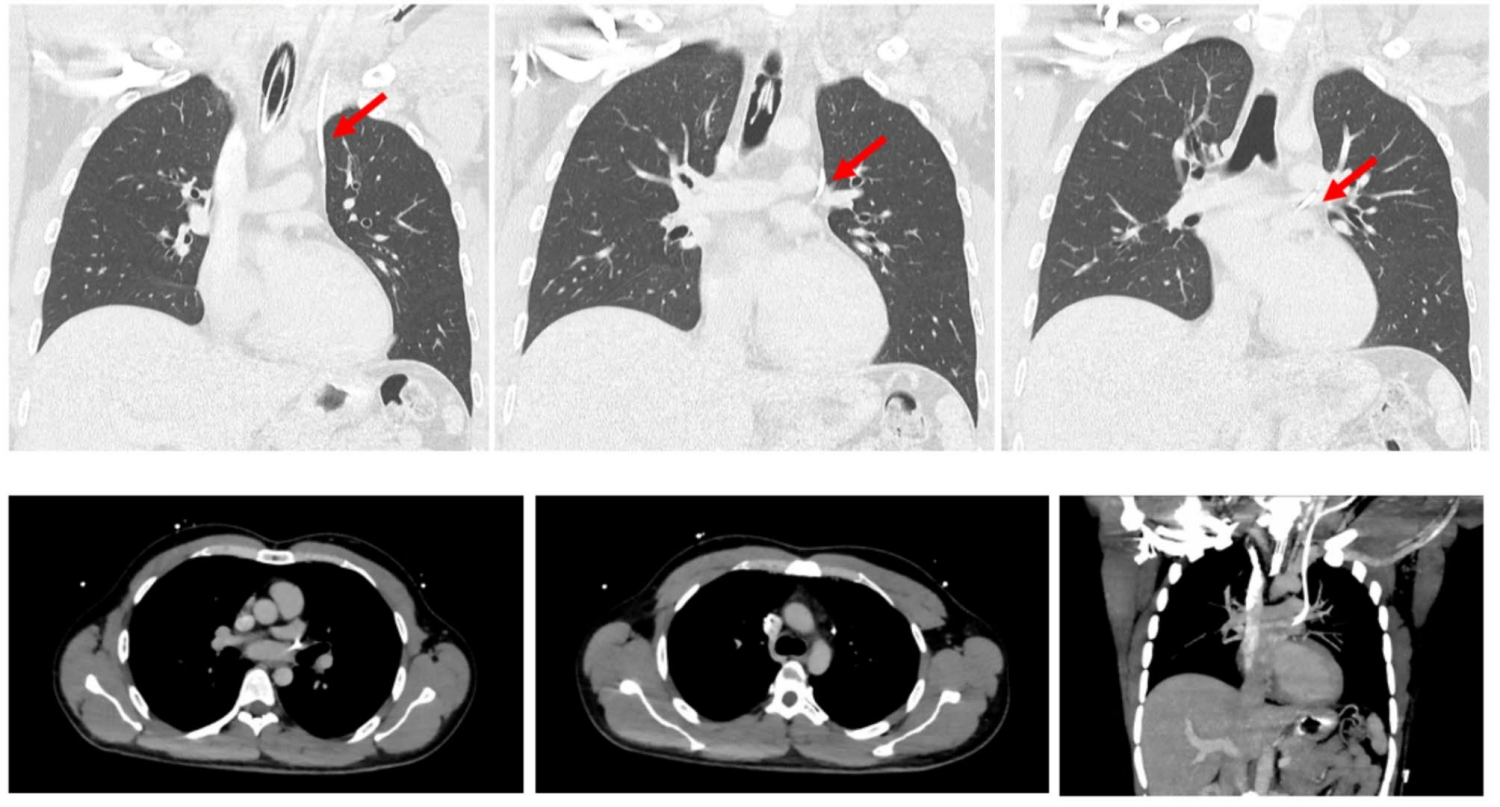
Position of the left sided central line with drainage into the left upper pulmonary vein shown in patient A’s CT scan (both lung window and maximum intensity projection)
